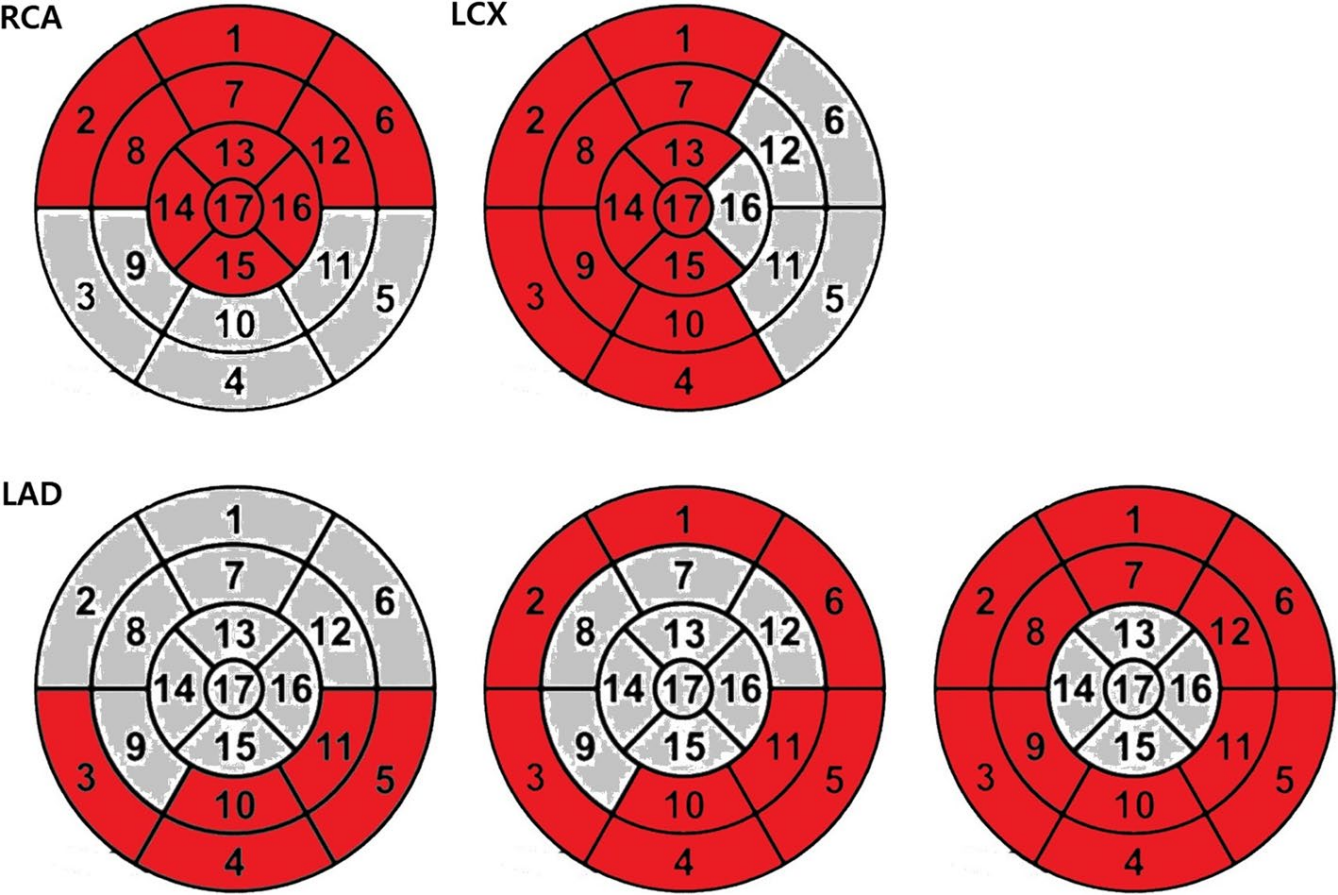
Example of bull's-eye polar map showing the typical coronary artery distribution established by the American Society of Echocardiography and European Association of Cardiovascular Imaging [1]. The segments that could show RWMA were marked in grey, while those without RWMA were marked in red. RCA, right coronary artery; LCX, left circumflex coronary artery; LAD, left anterior descending artery; RWMA, regional wall motion abnormality
